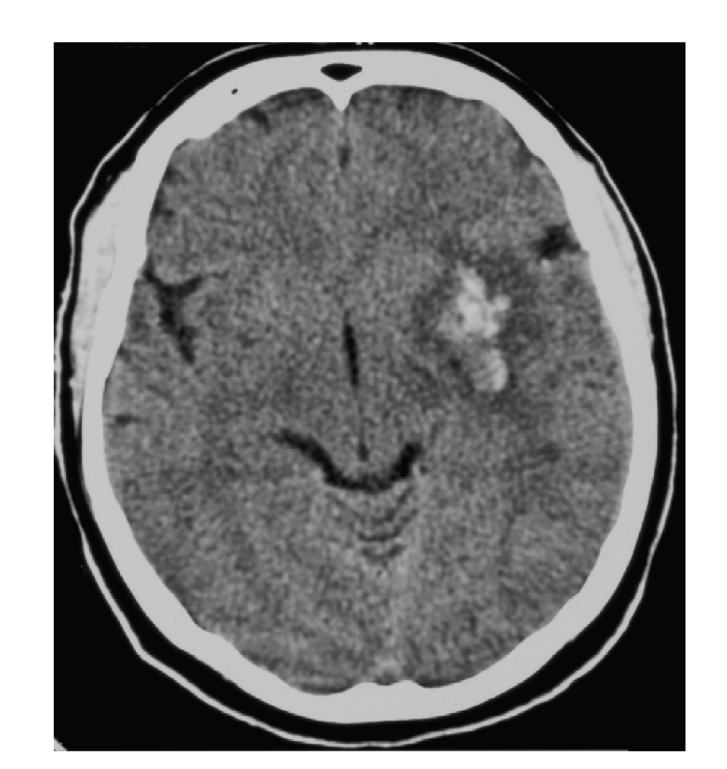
Control CT scan shows a small haematoma in the left hemisphere.
